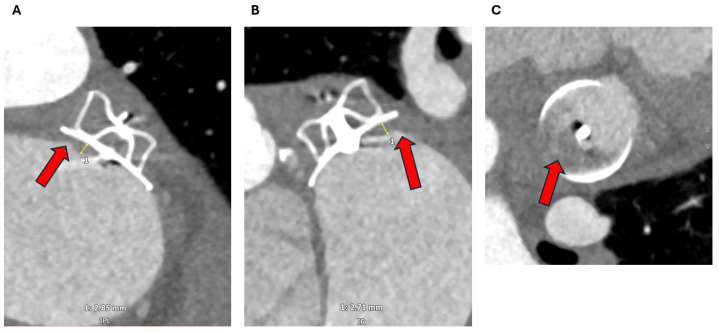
A 72-year-old gentleman imaged with cardiac CT post-LAAO with an Amplatzer Amulet device. A laminar hypoattenuated thickening (HAT) (red arrows) partially covers the antero-superior aspect of the device disc (A–C) and is continuous with the LA endothelium. It measures 3 mm at its maximum thickness (A,B). These features are in keeping with a low-grade HAT and likely represent the endothelialisation process. CT: Computed tomography; LA: Left atrium; LAAO: Left atrial appendage occlusion.
